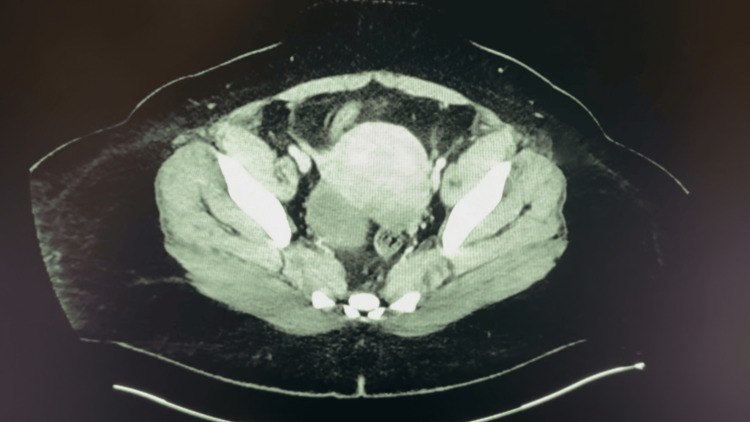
CT imaging of Patient A’s pelvis depicting an enlarged right ovarian cyst measuring 3.1 cm, furthering the diagnosis of ovarian torsion for Patient A.
